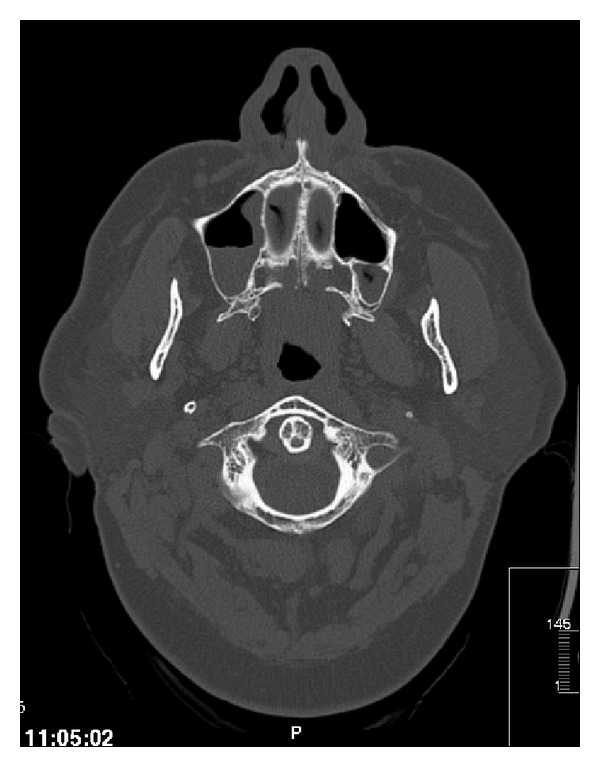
Computed tomography scan of the orbits.
